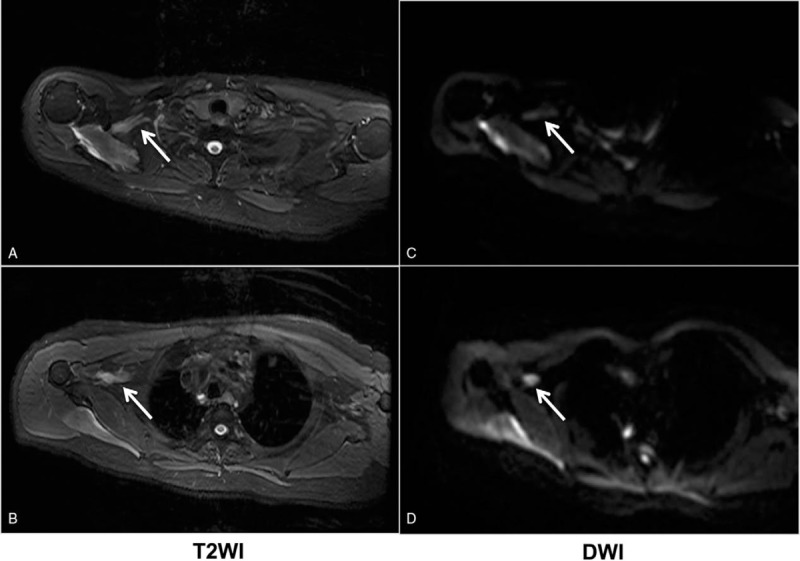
MR images showed the right brachial plexus and axillary nerves had hypersignal intensity in T2WI (A-B); the involved nerves were also hyperintense in DWI (C-D).
